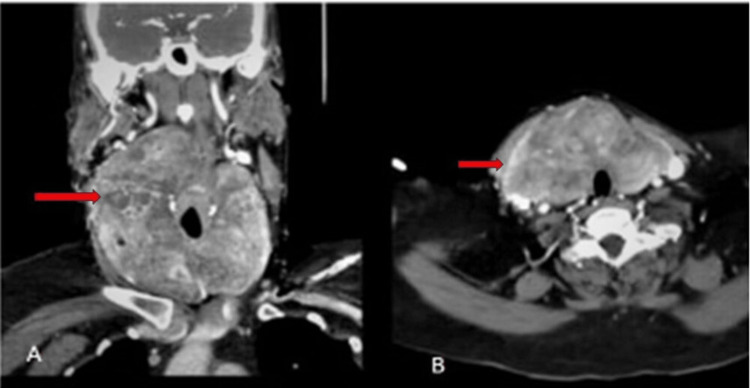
CT showing enhanced thyroid mass lesion. (A) Coronal CT showing heterogeneous enhanced thyroid mass lesion (red arrow) along with compression of the pharynx. (B) Axial CT also showing enhanced thyroid mass lesion (red arrow) along with compression of the pharynx, and the trachea is slightly deviated to the left side.
